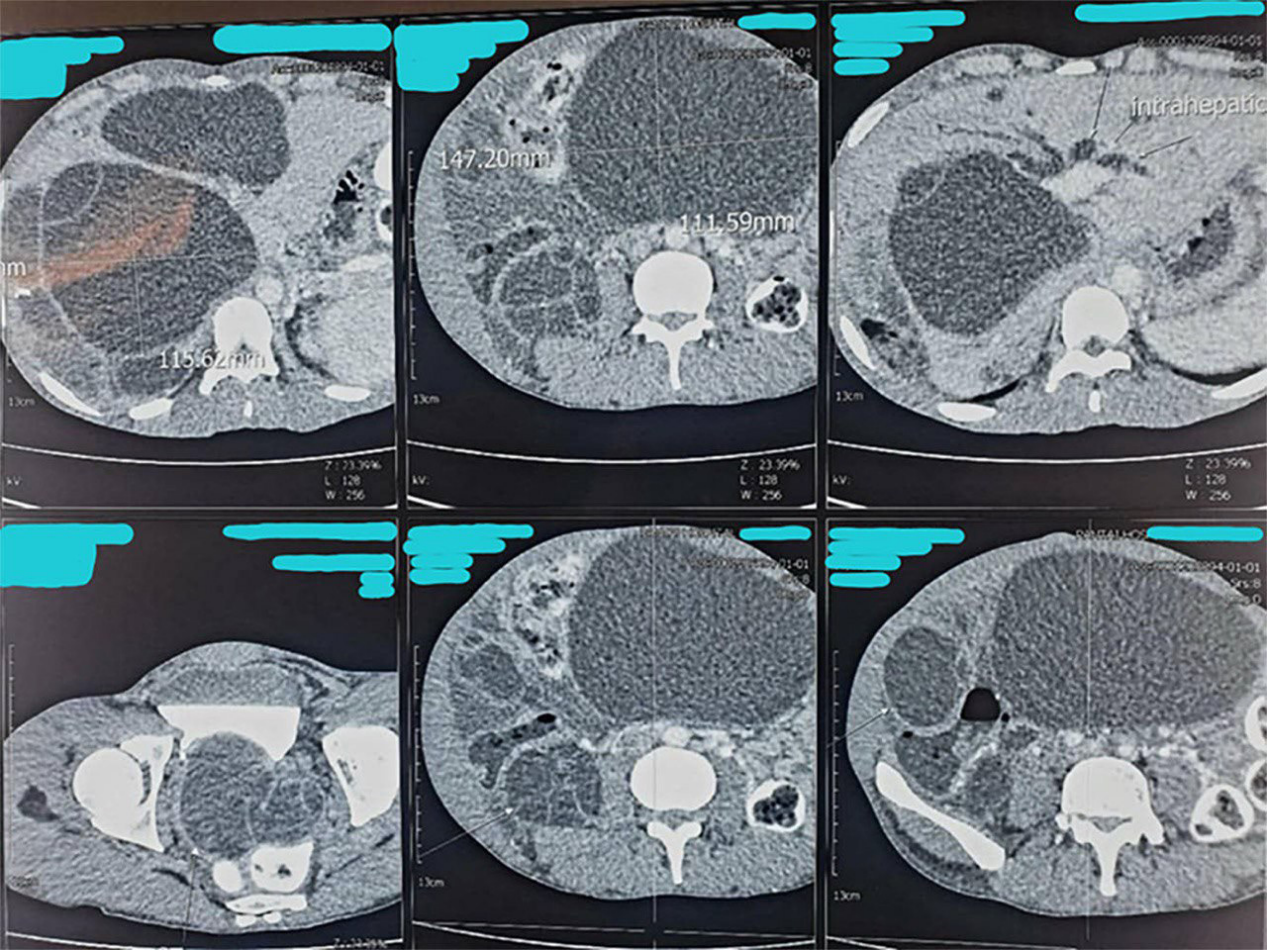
Contrast-enhanced CT scan of the abdomen showing multiple upper axial sections of the liver with a large intrahepatic hydatid cyst. The cyst measures approximately 111.59 mm × 147.20 mm at its maximal dimensions. The slices demonstrate the extent of the cyst within the liver parenchyma.
